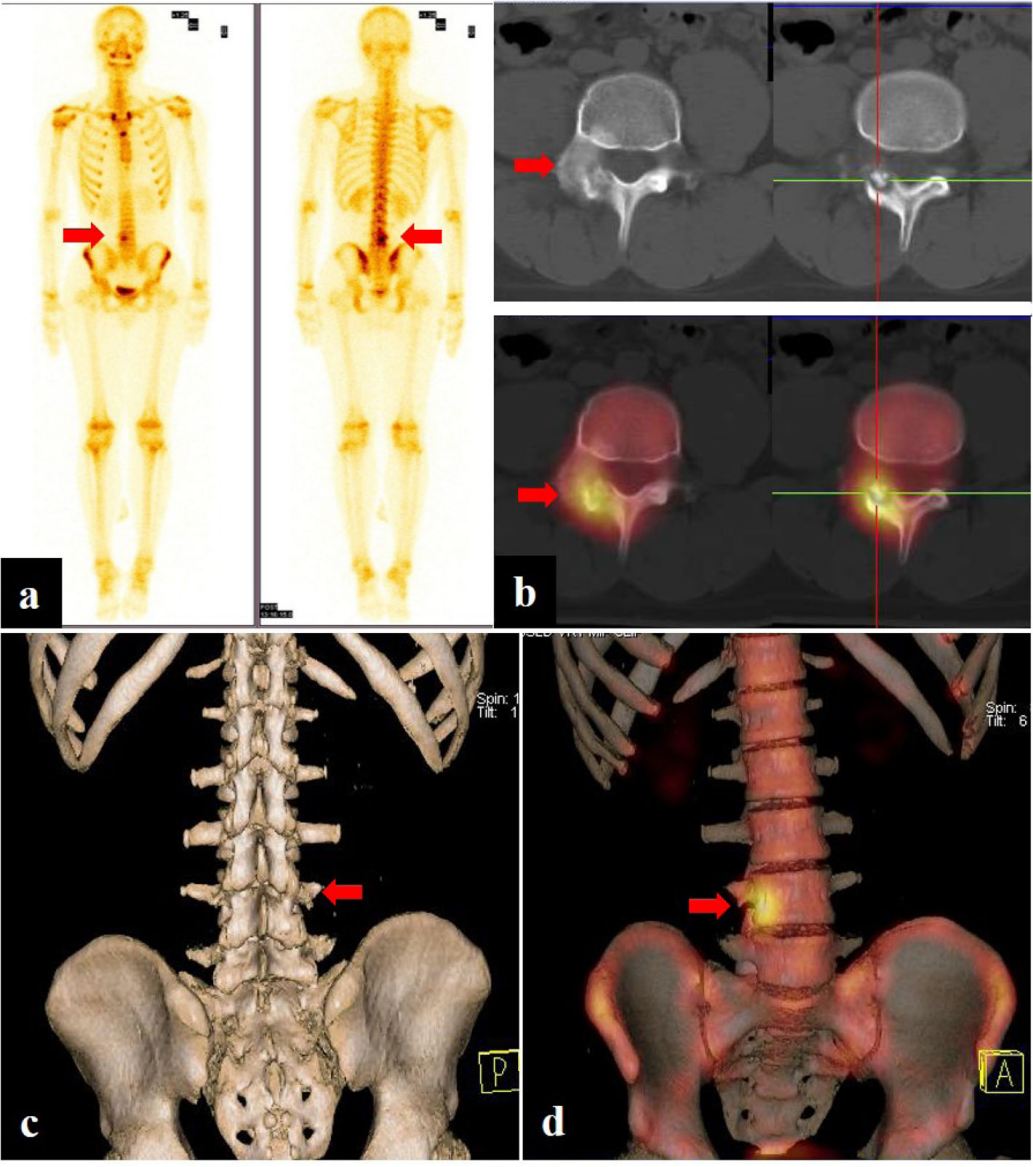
Patient no. 17, male, 28 years old. Anteroposterior (a) and lateral (b) radiograph demonstrated bone destruction and hyperplasia in the C3–4 level (yellow arrow). CT (c) and MRI (enhanced T1W/FS and T2W sequence) (d, e) of the cervical spine showed a 20.0-mm lesion on the right transverse processes and laminae of C3–4, a typical osteogenic feature of OB (orange arrow). Planar bone scan displayed high uptake around C3–4 cervical vertebral body (g), whereas SPECT/CT  (f) and 3D reconstruction images showed more details on the lesion and provided more information for orthopedist (red arrow)
